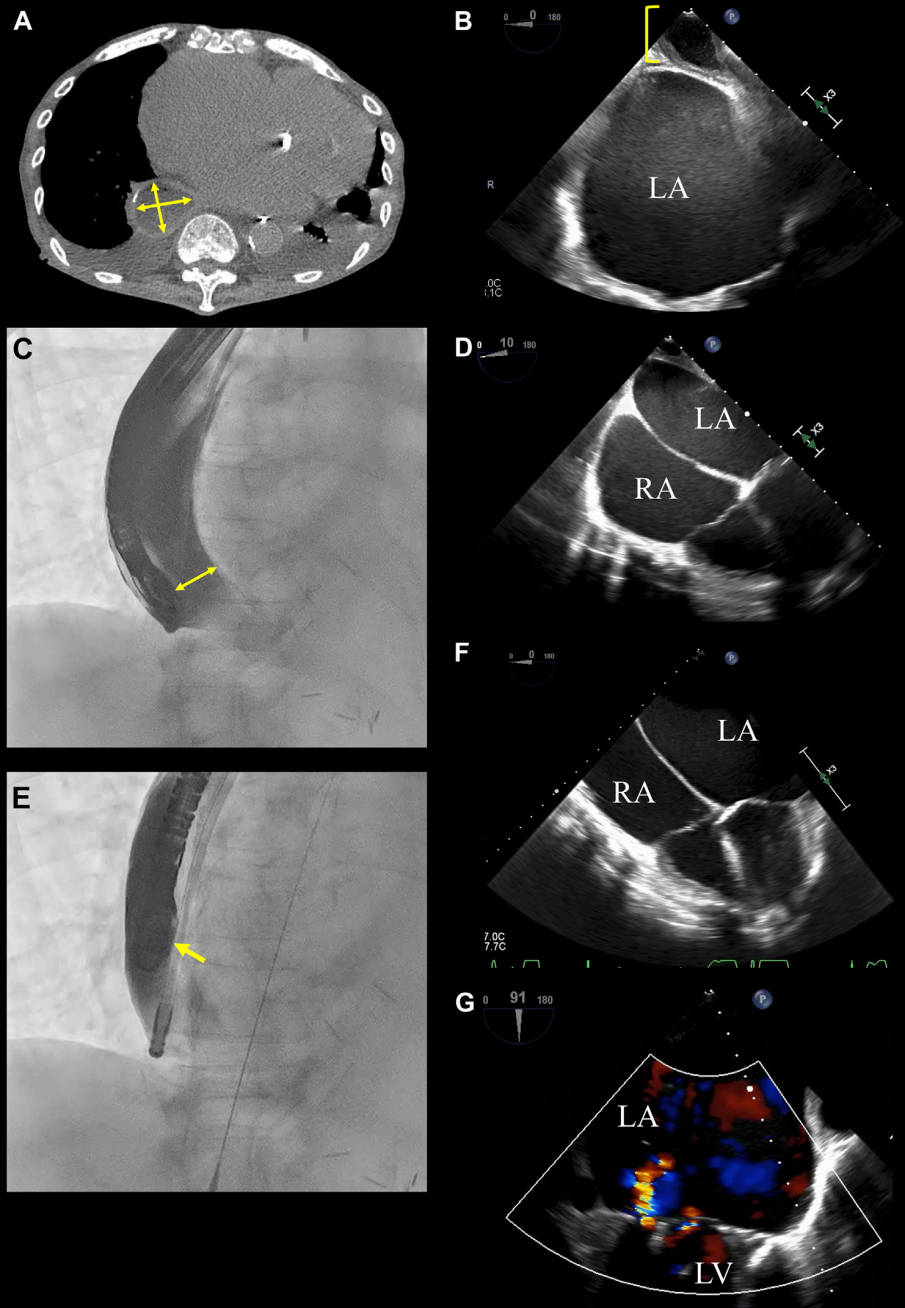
Visualization and Management of Severe Esophageal Achalasia During Transesophageal Echocardiography for Mitral Valve Transcatheter Edge-to-Edge Repair (A) Computed tomography shows significant dilation of the midesophagus approximately 4 × 3.5 cm in diameter (yellow arrows). (B) Transesophageal echocardiography during probe insertion demonstrating approximately 2.5 cm of residual esophageal contents located between the heart and esophageal wall, despite adherence to fasting protocols. (C) Fluoroscopic image obtained during esophageal contrast imaging. The image reveals substantial dilation of the midesophagus measuring approximately 4 cm, with a 2.5-cm gap between the probe and esophageal mucosa (yellow arrow). (D) Predecompression transesophageal echocardiographic image in the 4-chamber view shows the presence of residual esophageal contents, reducing the clarity of mitral valve visualization. (E) Fluoroscopic image after decompression using a gastric tube. The distance between the probe and esophageal mucosa has been reduced to nearly zero, indicating successful decompression (yellow arrow). (F) Postdecompression transesophageal echocardiographic image in the 4-chamber view shows the absence of residual esophageal contents, leading to clearer and improved visualization of the mitral valve. (G) Transesophageal echocardiographic image obtained after mitral valve transcatheter edge-to-edge repair, showing a significant reduction to mild-to-moderate mitral regurgitation. LA = left atrium; LV = left ventricle; RA = right atrium.
